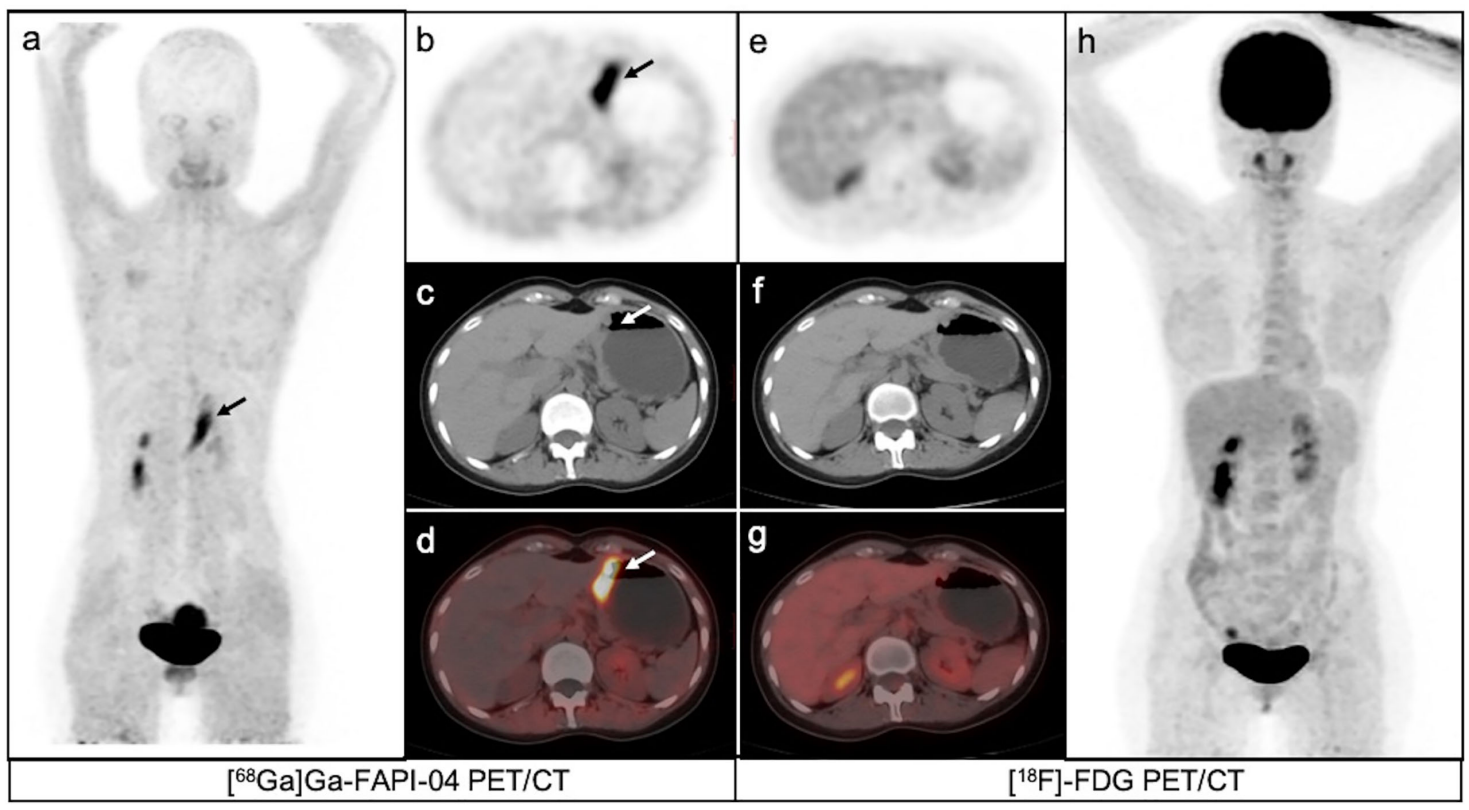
A 35-year-old female patient with moderately differentiated gastric adenocarcinoma based on pathological biopsy under gastroscopy. [^68^Ga]Ga-FAPI-04PET/CT (A–D) revealed high uptake in the primary tumor (arrows, SUVmax=8.3) but negative uptake was observed on [^18^F]-FDG PET/CT (E–H).
